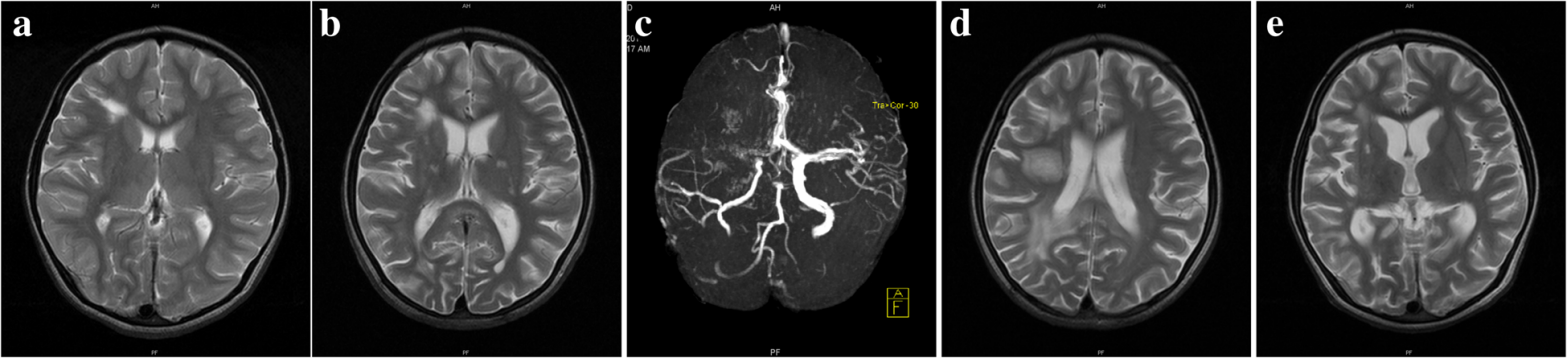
The brain MRI findings of a patient affected by CNS vasculitis and stroke. a A baseline MRI image showing T2 hyperintensities involving the right frontal white matter and basal ganglia. b Analysis performed following the diagnosis of CNS vasculitis, demonstrating multiple high signal intensity lesions in the periventricular deep and subcortical white matter. c The characteristic appearance of moyamoya phenomena-induced vasculopathy is noted. d Nine days later, following the development of stroke symptoms, additional changes consistent with white matter demyelination were identified. e Three months later, there were improvements in the high signal intensity lesions and no recurrences of infarction
